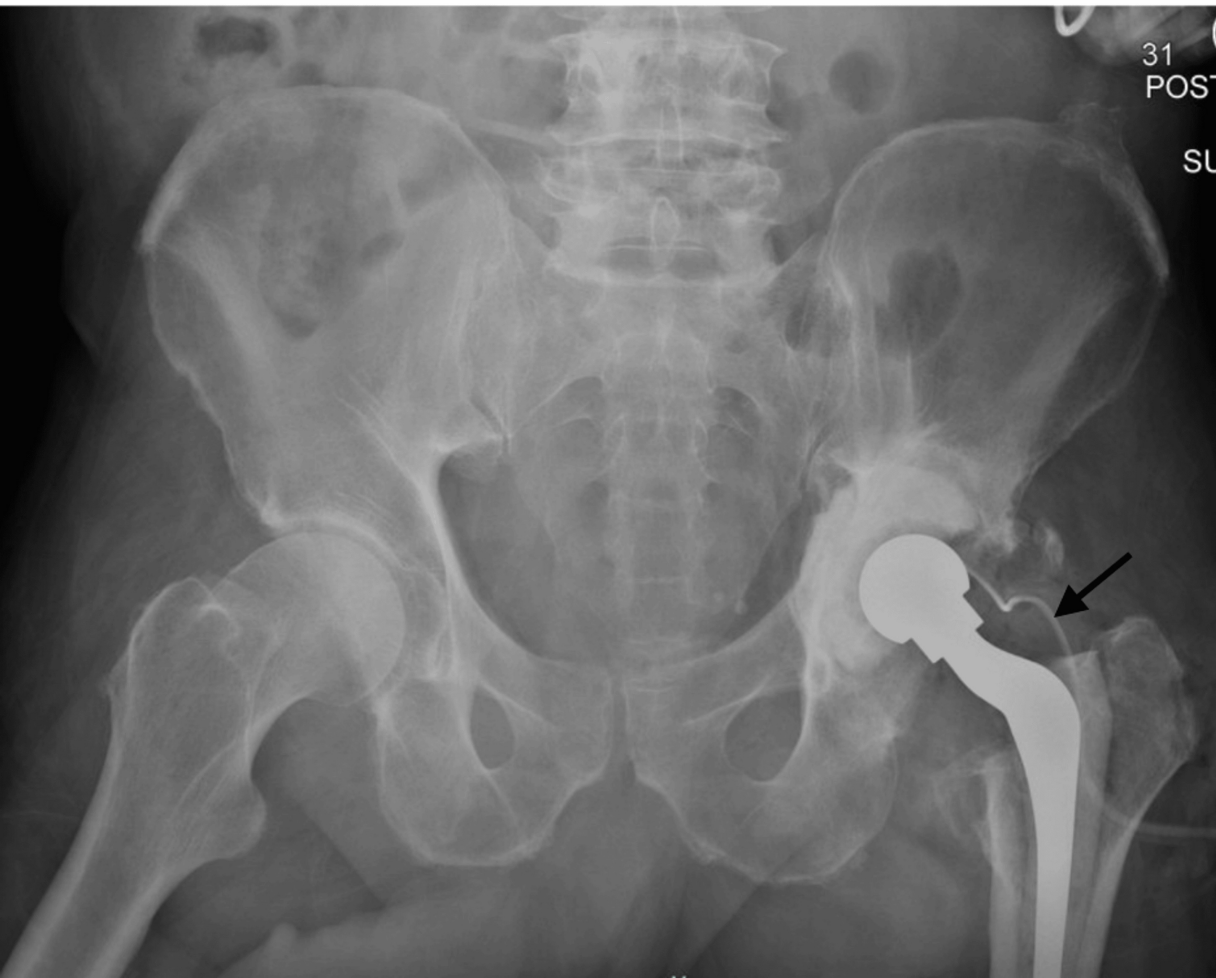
Postop-operative AP pelvis radiograph Demonstrates a DePuy Prostalac cemented polyethylene cup, size 4 + 13 mm offset Prostalac stem and size 32 mm Articul/eze™ metal head, and visible drain.
